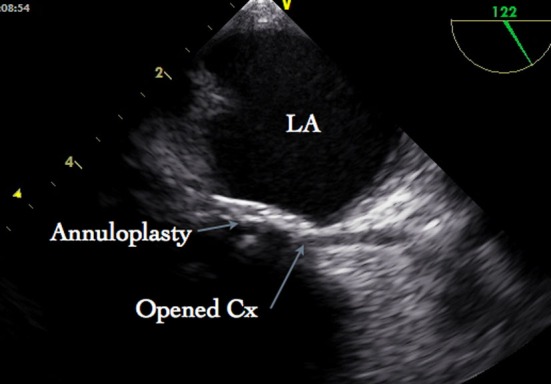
Transesophageal echocardiography after corrected procedure. Circumflex artery (Cx) is opened. LA, Left atrium.
